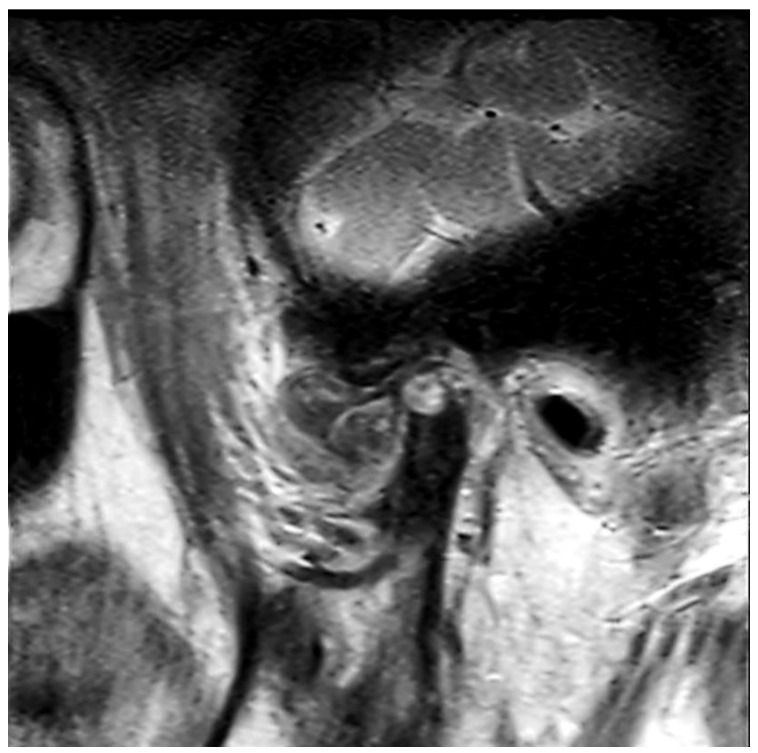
Magnetic resonance image. Proton density-weighted image of the sagittal plane.
